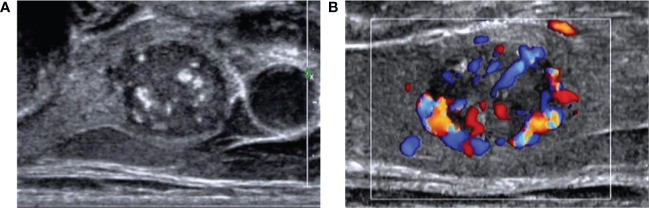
Images of a 55-year-old man with medullary thyroid carcinoma (MTC) (A) Ultrasound image showed a solid hypoechoic nodule with a clear boundary and lobulated margin. The aspect ratio was <1, and coarse calcification could be seen in the nodule. (B) Color Doppler flow imaging showed abundant blood flow around and inside the nodule.
